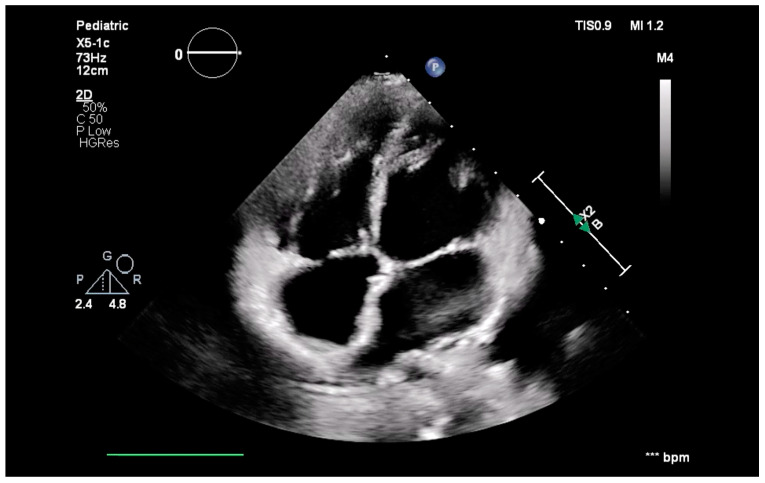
The last echocardiography, performed 1 year postoperatively; 4 chamber view.
